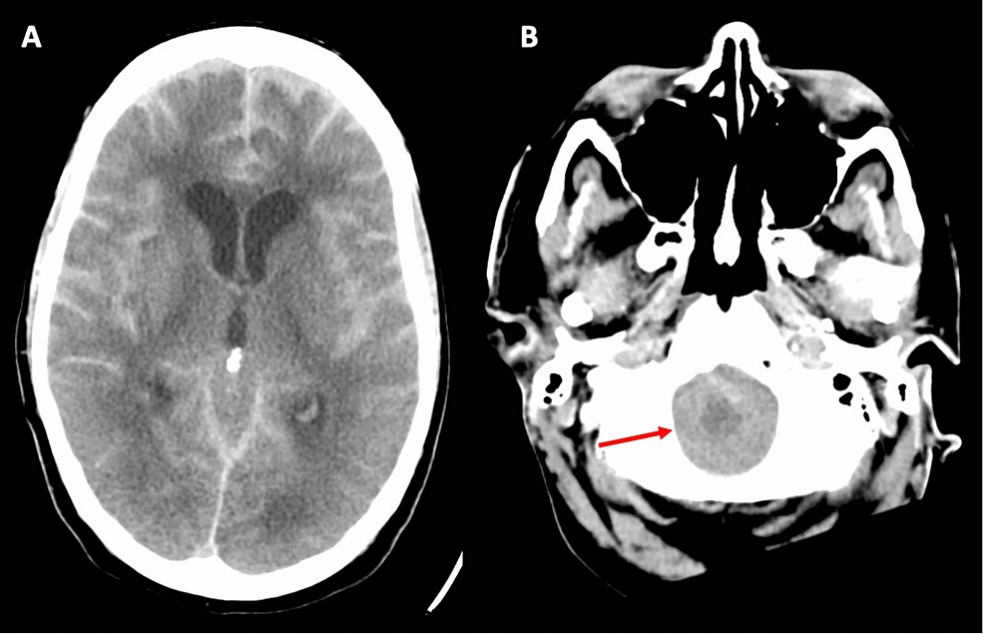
Final CT findings (A) Repeat CT (axial section) of the head with contrast after 15 hours showing decreased gray-white matter differentiation and fading intensity of the subarachnoid hyperintensity. (B) Repeat CT (axial section) of the head with contrast after 15 hours showing cerebellar tonsillar herniation through foramen magnum (red arrow).
